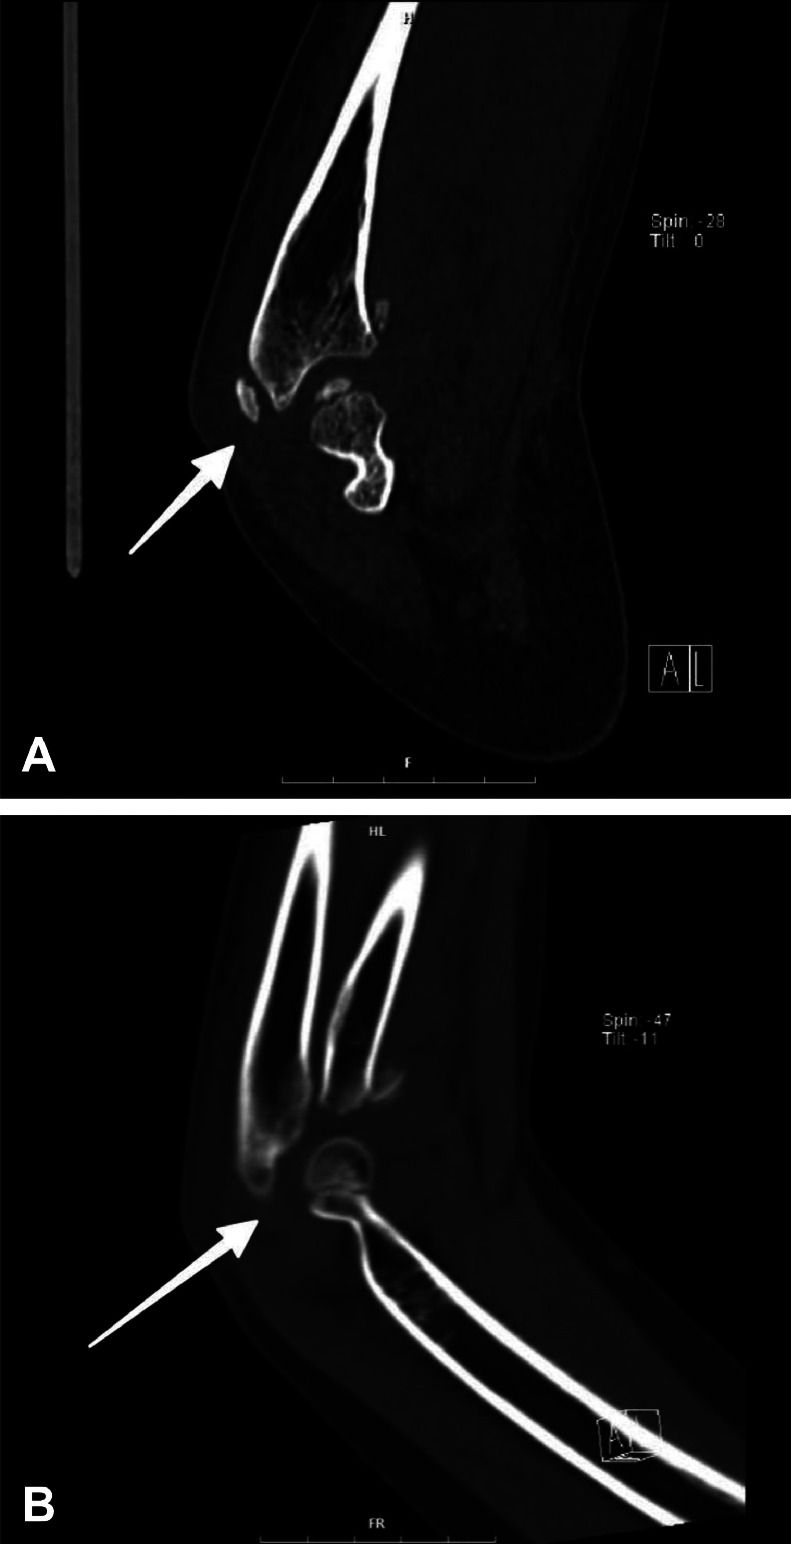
A: Axial CT image of the right elbow Computed tomography in axial plane, bone window, at the level of the radial head, demonstrating the comminuted radial head fracture (arrow) with multiple fragments. Slice thickness: 0.75mm, with 130 kVp settings. B: Sagittal CT image of the right elbow Computed tomography in the sagittal plane and bone window demonstrates the coronoid process fracture (arrow) and posterior subluxation of the radius and ulna. This image clearly shows the Type II coronoid fracture according to the Regan-Morrey classification.
